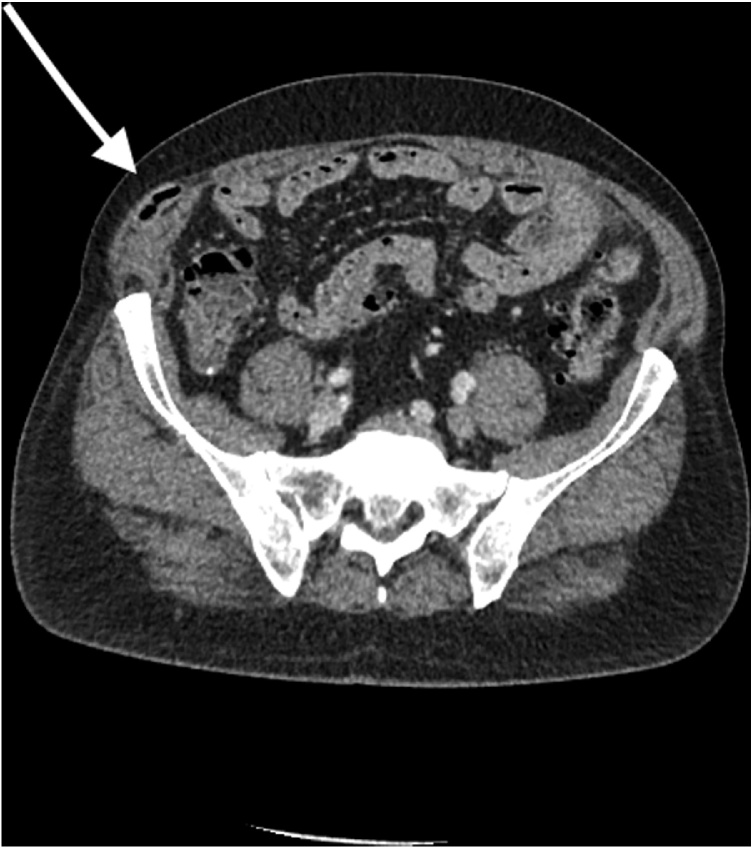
CT Scan showing Spigelian hernia containing the appendix (arrow).
